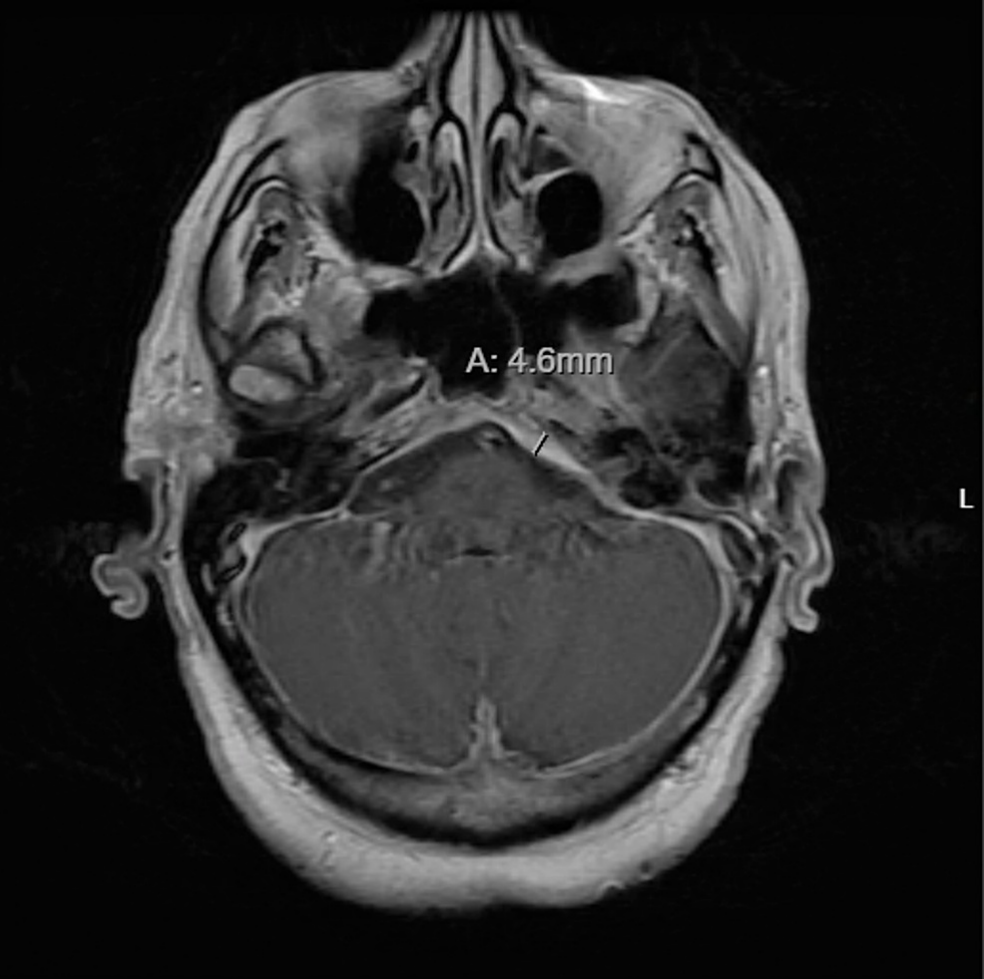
Brain MRI T1-weighted axial section showing thickening of the left clivus.
